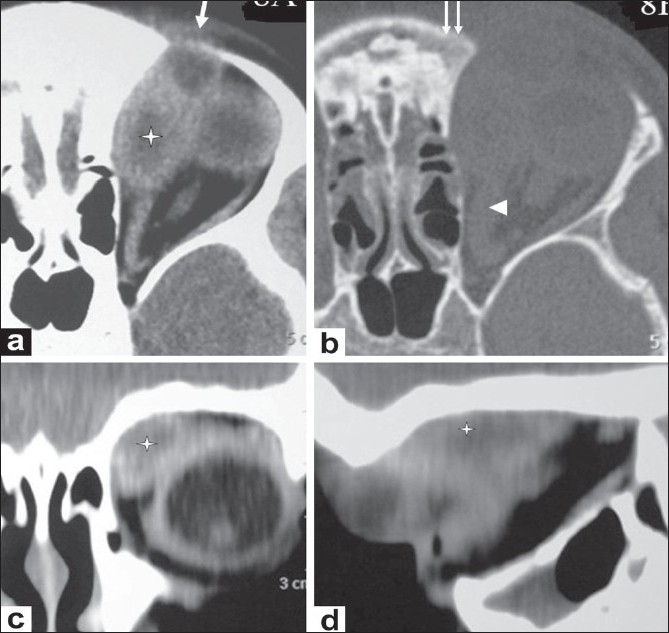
A 5-year-old boy presented with painless swelling (proptosis) of the left eye. Axial (a,b), coronal (c) and sagittal (d), CT scans show an extraconal orbital abscess (asterisk in a,c,d) in the superomedial region of the left orbit with extension into the preseptal tissue (arrow in a). The adjacent medial rectus muscle appears slightly thickened (arrowhead in b). The adjacent bone shows scalloping, and there is a focal area of subtle loss of definition of the cortical margin anteromedially (double arrow in b).
